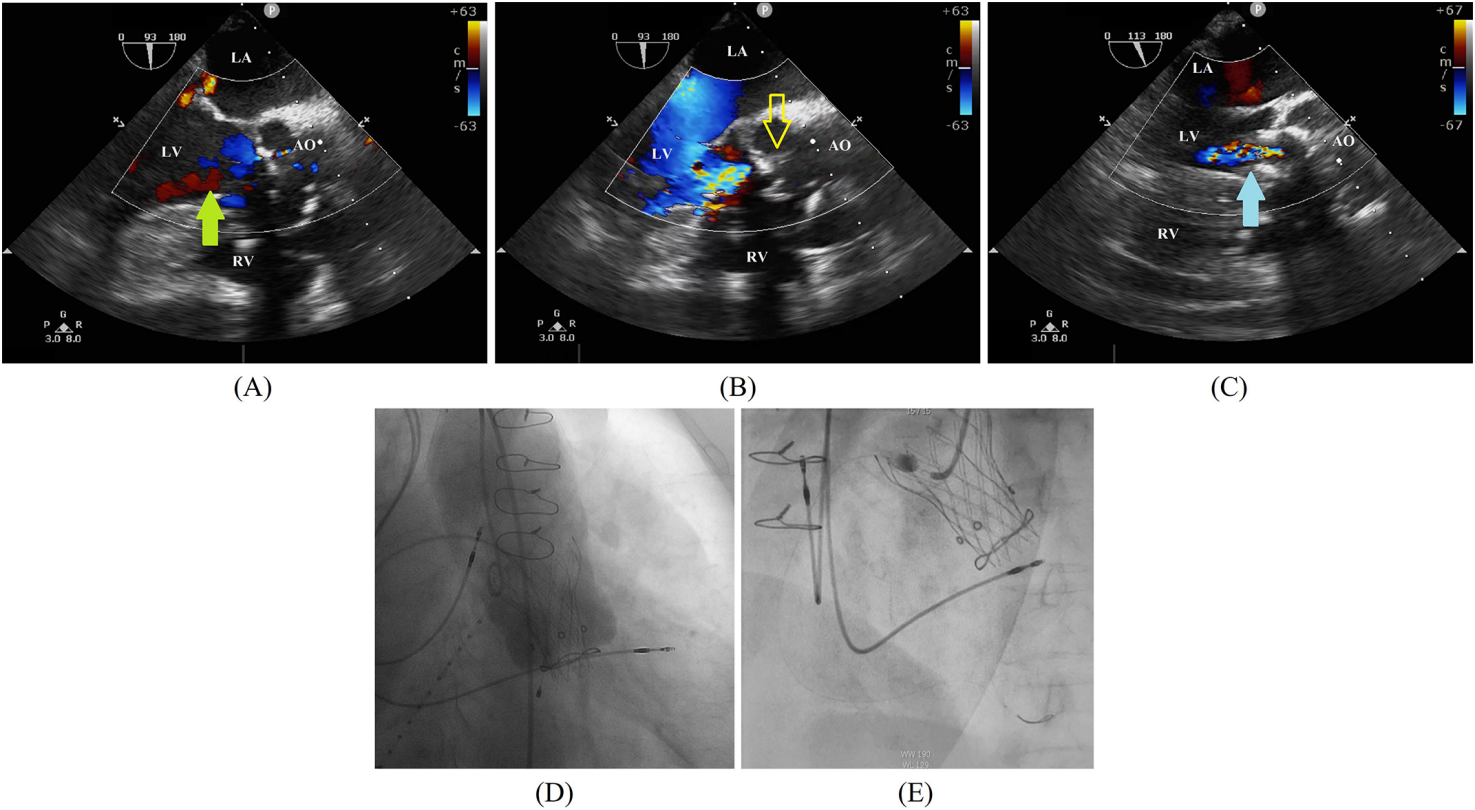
(A,B) A preoperative transesophageal echocardiogram demonstrates left coronary sinus perivalvular leakage (green arrow) and a thickened leaflet (yellow outlined arrow). (C) A postimplantation transesophageal echocardiogram demonstrates the absence of paravalvular regurgitation. A self-expanding valve bottom skirt fits left ventricular outflow to eliminate perivalvular regurgitation (blue arrow). (D) A final angiogram further confirms the absence of valvular or paravalvular regurgitation. (E) Coronary angiography demonstrates an obstruction of the proximal RCA. AO, ascending aorta; LA, left atrium; LV, left ventricular; RV, right ventricular.
